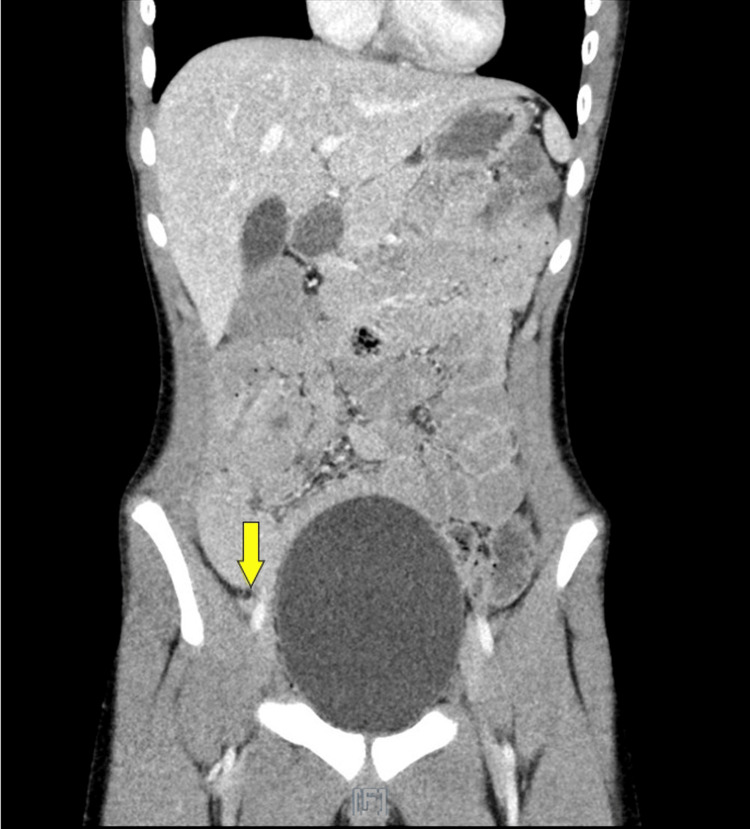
CT abdomen showing retrocecal appendicitis along diverticulae
